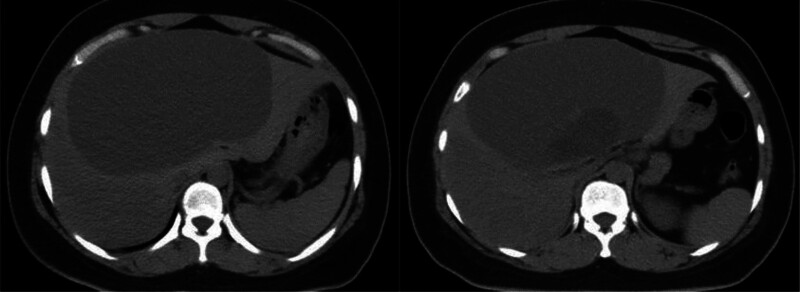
Abdominal CT showing a large multilocular cystic mass with septal enhancement in liver segment IV. CT = computed tomography.
